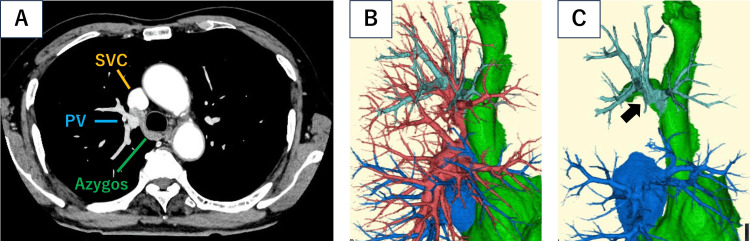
Partial anomalous pulmonary venous connection in the right upper lobe. A: Contrast-enhanced computed tomography (SVC: superior vena cava; PV: pulmonary vein; Azygos: azygos vein) B: Contrast-enhanced computed tomography-based three-dimensional reconstruction (red: pulmonary artery; blue: pulmonary vein; light blue: pulmonary vein of the upper lobe that returns to the vena cava; green: azygos vein and superior vena cava). C: Contrast-enhanced computed tomography-based three-dimensional reconstruction (blue: pulmonary vein; light blue: pulmonary vein of the upper lobe that returns to the vena cava; green: azygos vein and superior vena cava).
